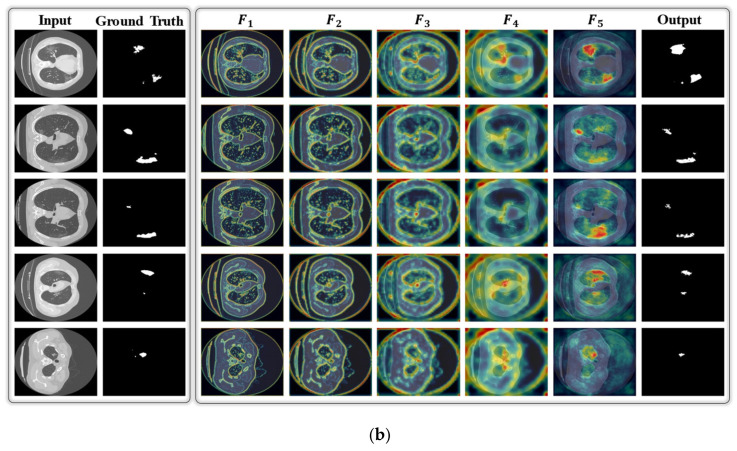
Visualization of multiple CAM outputs obtained from the different parts of the proposed DAL-Net for given data samples of the (a) COVID-19-CT-Seg data, (b) MosMed data.
